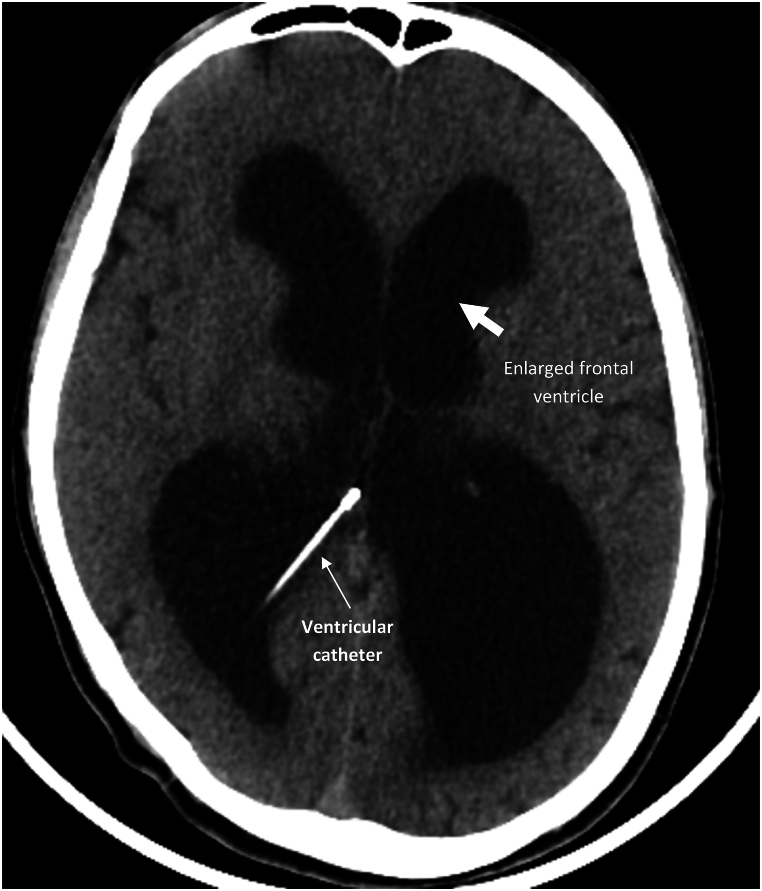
Axial views of CT scan showing the large size of the ventricles and hydrocephalus.
